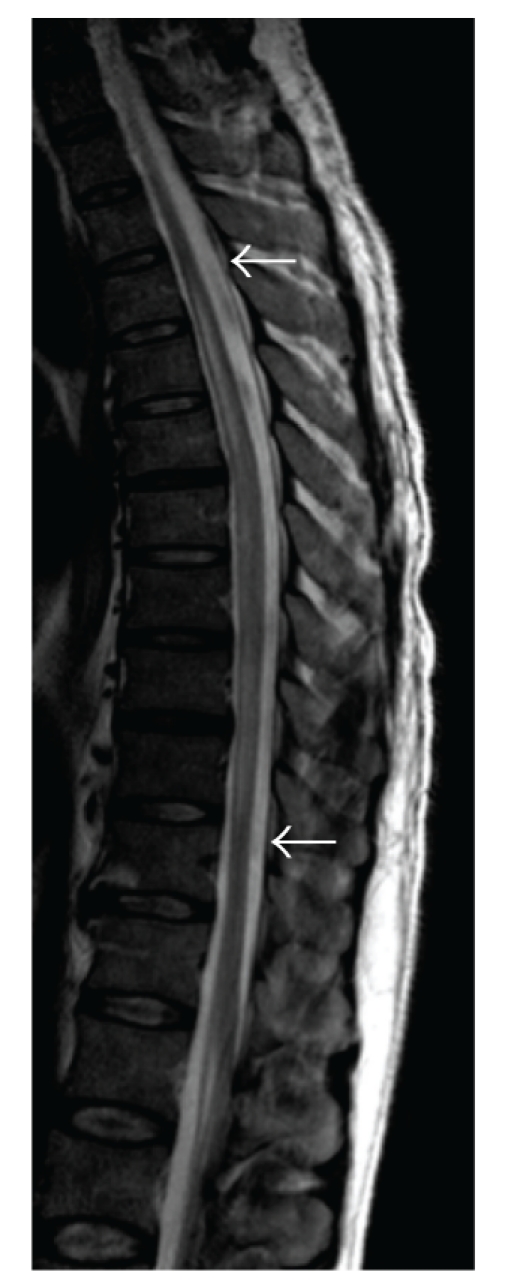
Sagittal T2-weighted spinal MRI shows an intramedullar hyperintense lesion extending from T4 to T10 (between the arrows).
